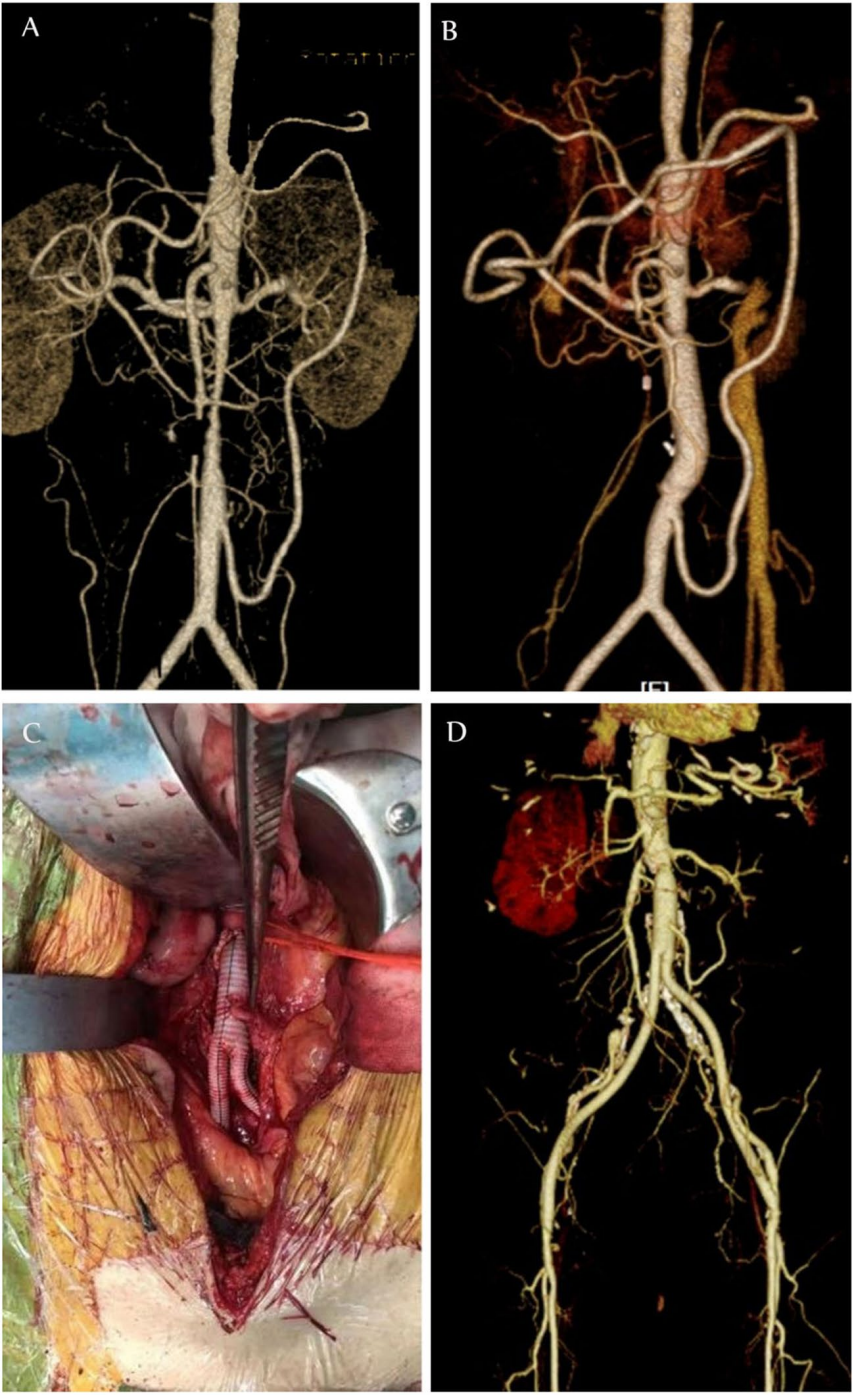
A Riolan arch in patients with abdominal aortic stenosis. B IMA is protected during abdominal aortic stent implantation. C IMA was anastomosed to artificial vessels. D CTA after abdominal aortic resection with artificial vessel replacement. IMA, inferior mesenteric artery; CTA, computed tomography angiogram
